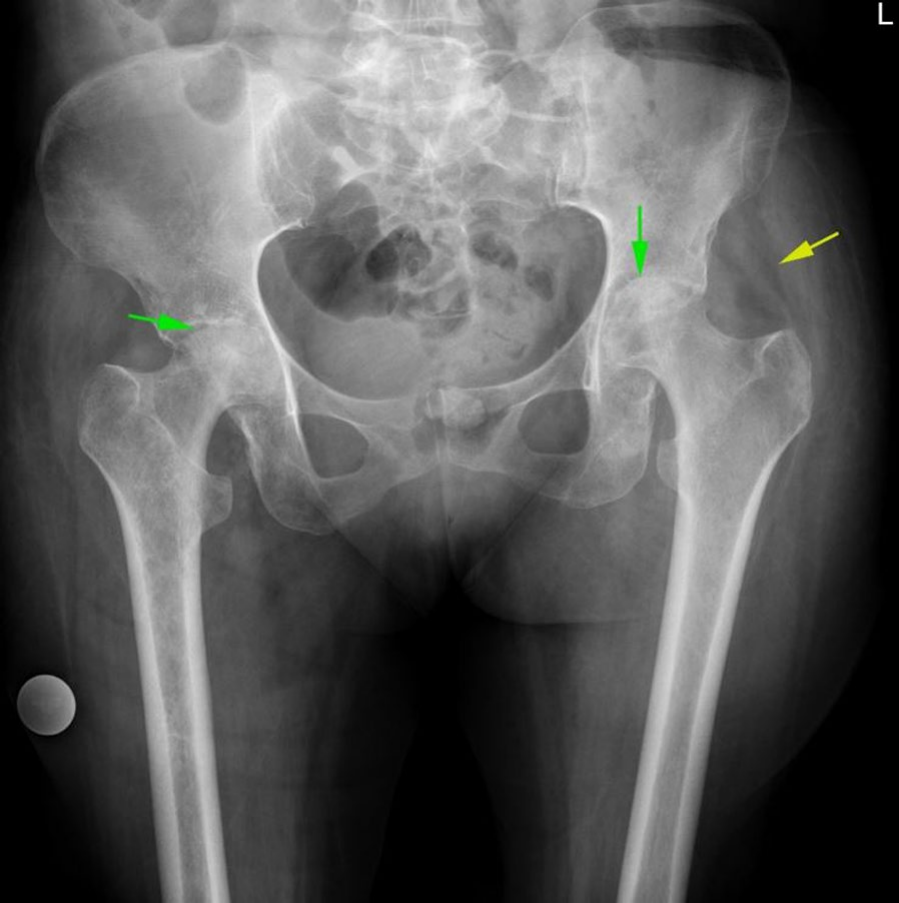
AP view pelvic X-ray shows sclerosis and collapse of bilateral femoral head in favor of femoral head AVN (green arrow). Soft tissue edema and displaced gluteal fat plans are in favor of joint fluid and possible inflammatory process (Yellow arrow)
